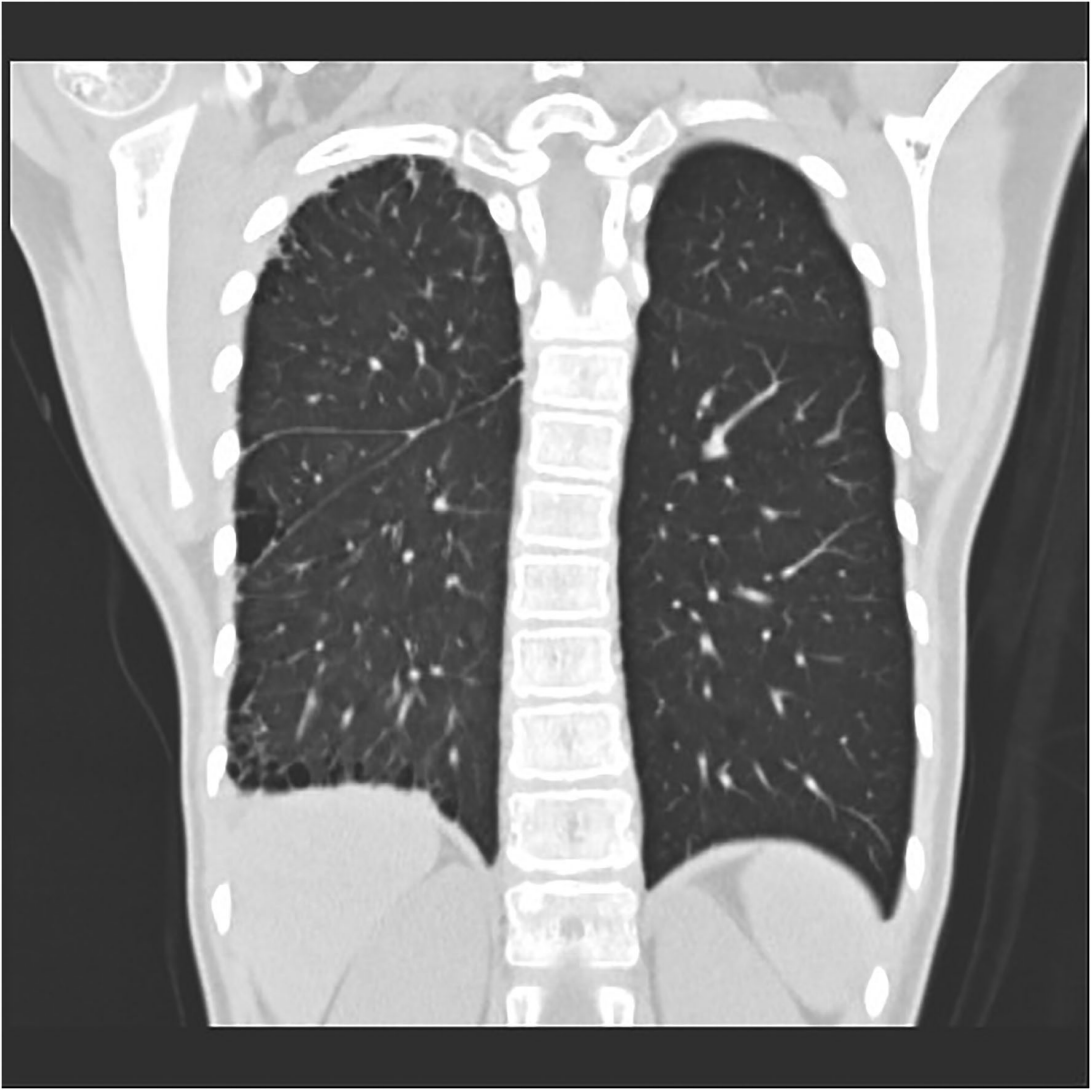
Right proximal interruption of the pulmonary artery in a 4-year-old female patient. A coronal view of a plain computed tomography scan shows a serrated pleural shadow in the right lung with a subpleural cystic lucency shadow.
